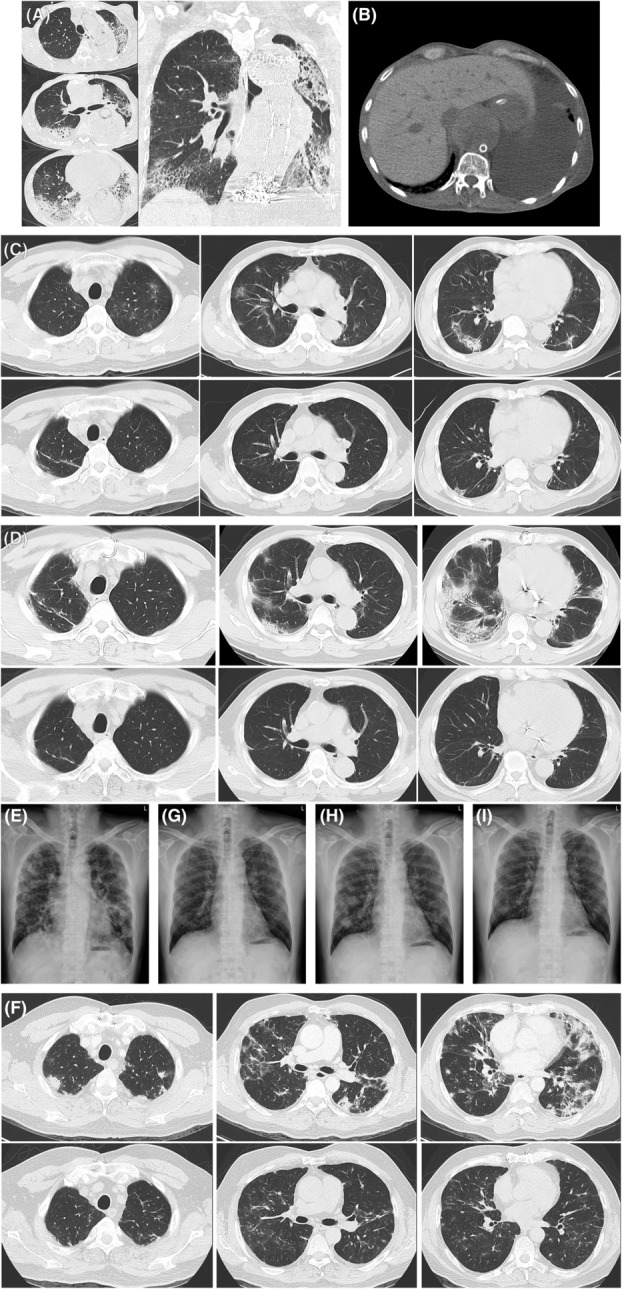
(A) Computed tomographic (CT) images of case 1 in transverse and coronal views. (B) Diffuse hyperintensity of the liver on the initial CT image of case 1. (C) Initial (upper 3 panels) and follow‐up (lower 3 panels) CT images of the first episode of interstitial lung disease (ILD) of case 2. (D) Initial (upper 3 panels) and follow‐up (lower 3 panels) CT images of the second (recurrent) episode of ILD of case 2. (E) The initial chest radiograph of case 3. (F) Initial (upper 3 panels) and follow‐up (lower 3 panels) CT images of the first episode of ILD of case 3. Serial subsequent chest radiographs of case 3, showing: (G) post‐treatment resolution of the first episode of ILD; (H) recurrent ILD following amiodarone re‐exposure; (I) resolution of the recurrent ILD.
